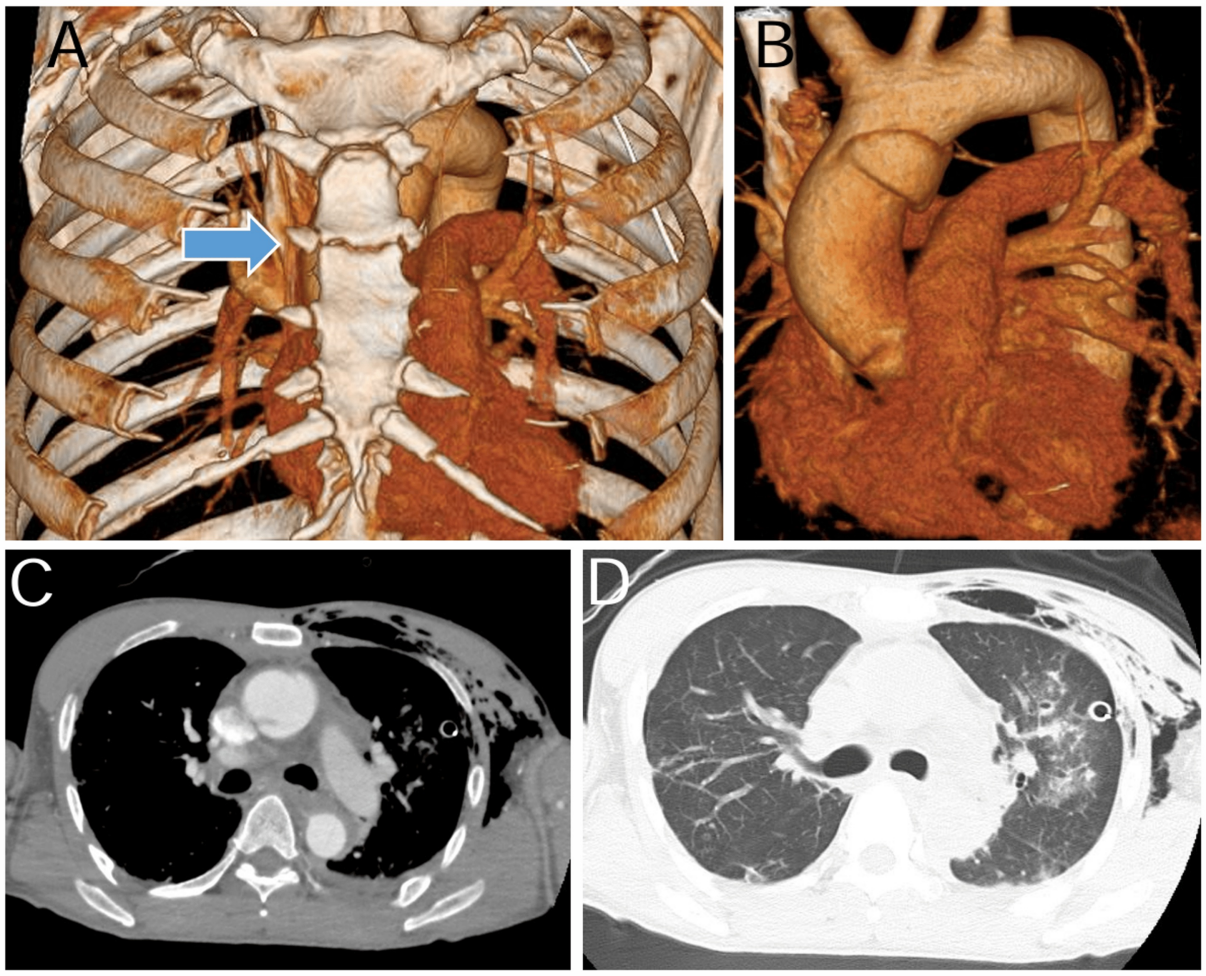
Preoperative contrast-enhanced computed tomography (A) Computed tomography (CT) reveals a sternal fracture; (B) The extent of the aortic dissection is localized with tears from the pulmonary artery to the posterior wall; (C) CT reveals acute ascending aortic dissection and coarse subcutaneous emphysema; (D) CT reveals pulmonary contusion and traumatic left hemopneumothorax Subsequently, a thoracic drain was inserted.
